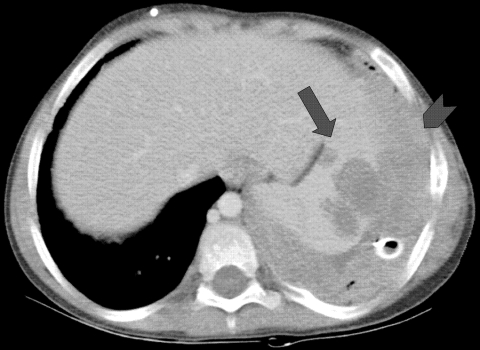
Computed tomographic scan showing infection with *Aspergillus viridinutans*, originating in the lungs, extending into the diaphragm (arrowhead), and producing hypodense splenic lesions (arrow).
